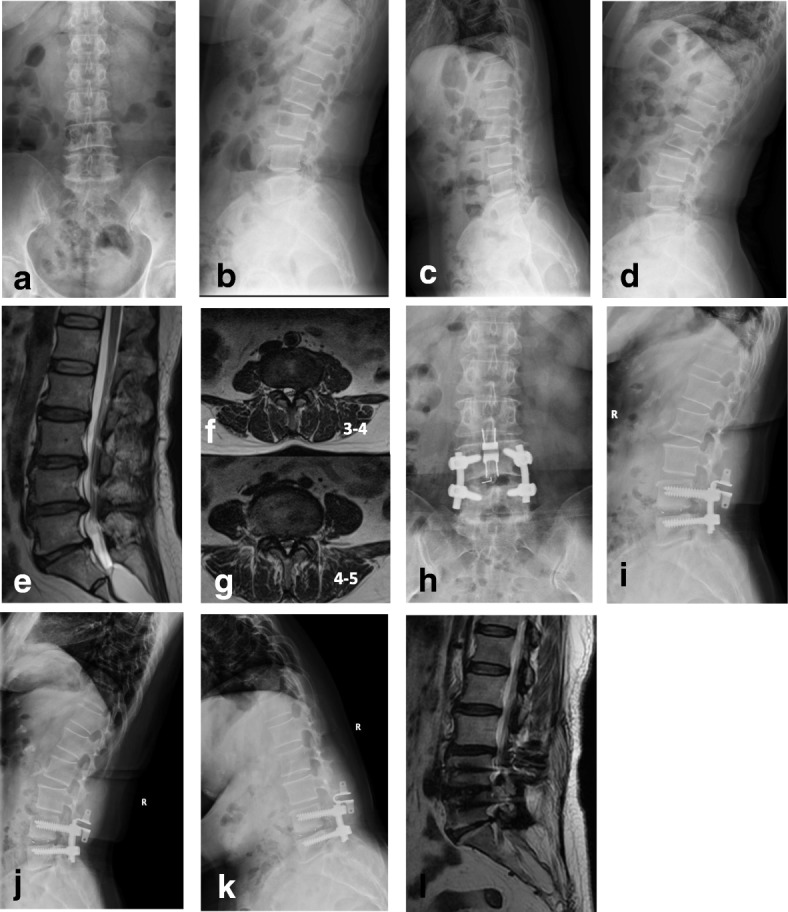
A 44-year-old, female, patient complained of lower back pain and pain in the left lower limb for 8 years, with aggravation for 6 months. This patient had spinal canal stenosis at L3–L5 and received Topping-off surgery. a–d X-ray for the lumbar spine in the anteroposterior/lateral and flexion/extension views before surgery; intervertebral mobility 3.9° at L2–L3 and 4.2° at L3–L4. e–g Lumbar MRI scan before surgery indicated spinal canal stenosis at L3–L5, with modified Pfirrmann grade of disc 4 at L2–L3. h–k X-ray for the lumbar spine in the anteroposterior/lateral and flexion/extension views at 36 months after surgery. There was no significant change in the intervertebral mobility at L2–L3, which was 4.2° after surgery; the intervertebral mobility at L3–L4 was decreased to 2.9° after surgery. l MRI scan at 36 months after surgery indicated that the modified Pfirrmann grade of disc was still 4 at L2–L3
